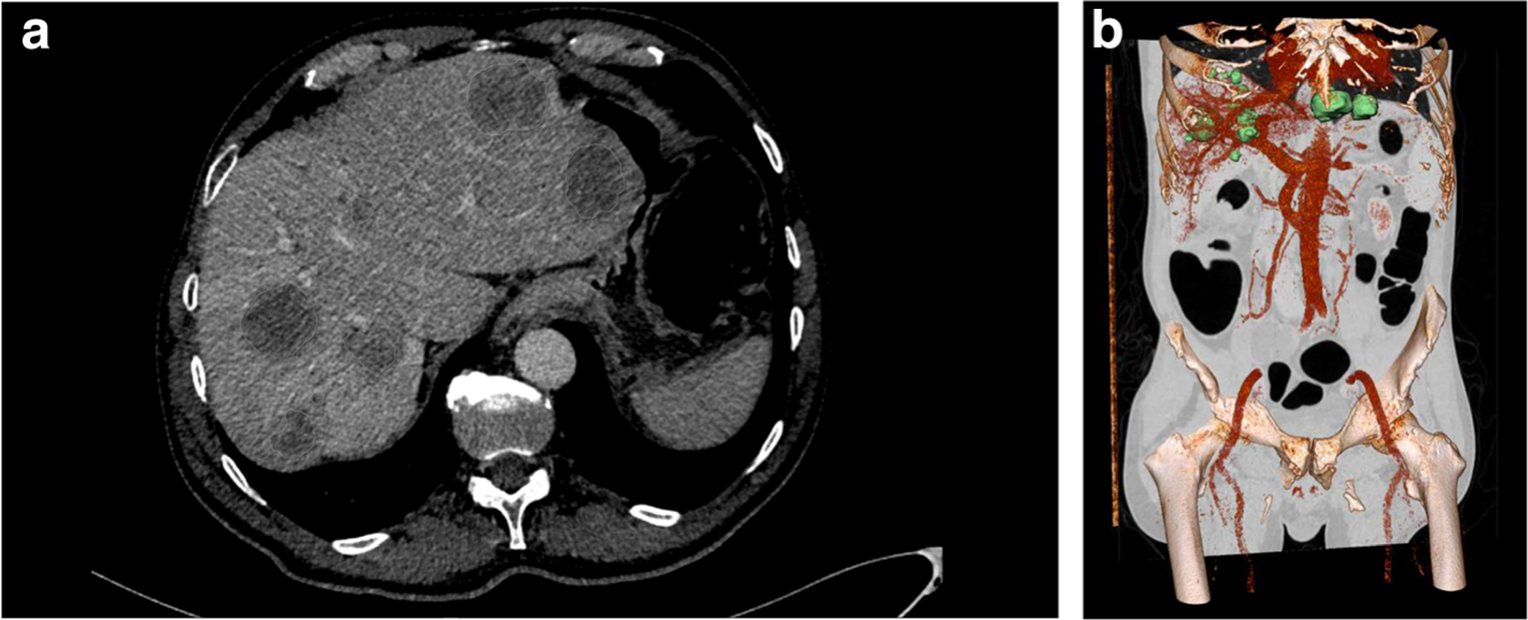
Example images from the DROID liver radiology dataset. (a) Axial CT slice of the upper abdomen with seven semi-automated segmented lesions. (b) Volume visualization of the whole abdomen with several segmented liver lesions in green. The bones and the portal vein are represented in 3D rendering mode for anatomical orientation. The grayscale image is presented on one coronal slice
